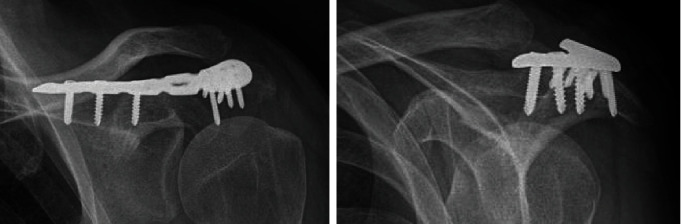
X-ray imaging two weeks postsurgery showing the usage of a lateral clavicle plate as a bridging plate for an acromion fracture.
